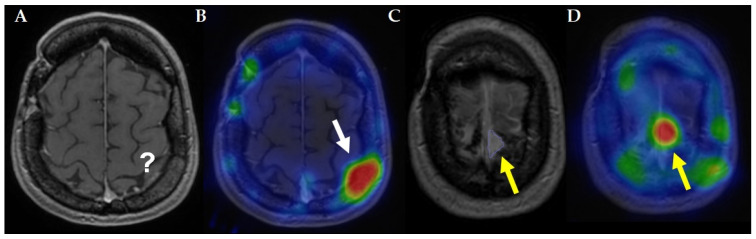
Representative comparison of contrast-enhanced MRI and somatostatin receptor-targeted SPECT in a patient with meningioma. (A) Contrast-enhanced MRI demonstrates no clearly detectable meningioma involvement of the cranial bone. (B) Corresponding SSTR SPECT image shows intense tracer uptake, indicating a somatostatin receptor-positive meningioma lesion (white arrow). (C) Contrast-enhanced MRI reveals an additional parafalcin foci in the precentral region, indicated by the yellow arrow. (D) SSTR SPECT confirms somatostatin receptor expression in the parafalcin lesion, demonstrating high tracer uptake at the corresponding site (yellow arrow). Images were acquired as part of routine clinical care and anonymized in accordance with institutional guidelines.
